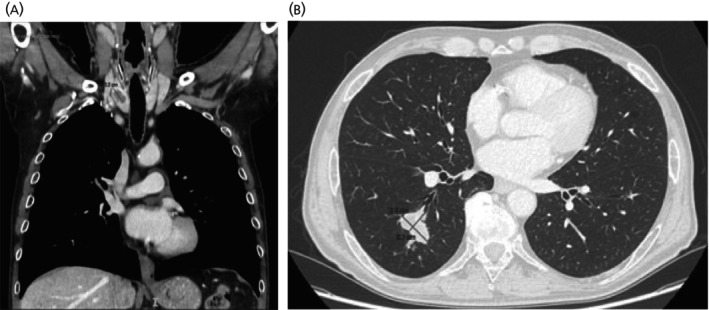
(A) CT scan of nodule with hypodense central component of the right thyroid lobe and (B) CT scan of hypodense lesion in the apical segment of the right lower pulmonary lobe.
